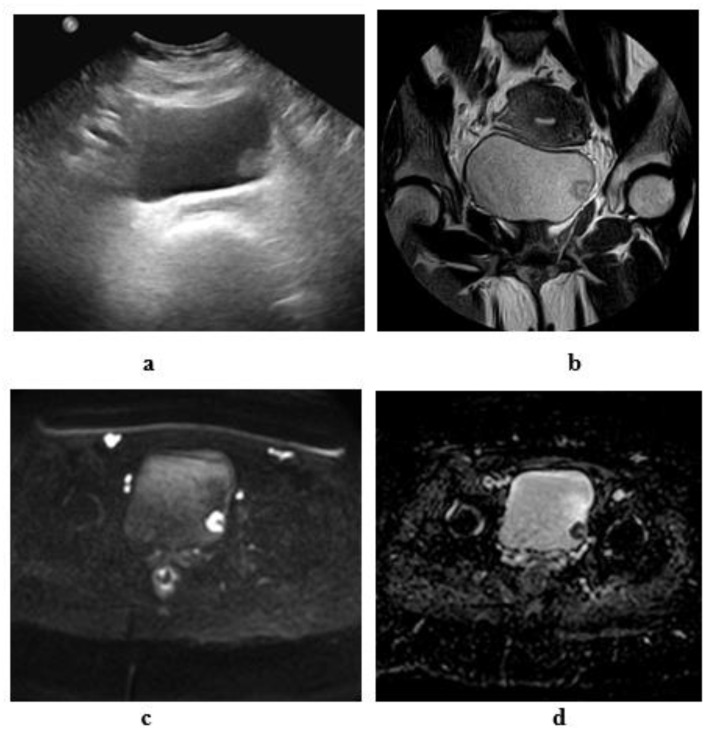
Stage T1 urothelial carcinoma: (a) transverse ultrasound image: an echogenic soft tissue mass at the left lateral wall. (b) A high resolution coronal T2 MRI: superficial soft tissue mass at the left lateral wall with arch- shaped high SI tumor covering the low SI submucosal stalk (inch worm sign). (c): DW-MRI (diffusion-weighted magnetic resonance imaging) (b value = 1400): showing restricted signals of the tumor and not the submucosal stalk. (d): ADC (apparent diffusion coefficient) map: the tumor displays hypointense SI and hyperintense SI of the submucosal stalk. Note the intact muscle wall of the urinary bladder. (ADC value = 1 × 10^−3^ mm^2^/s).
